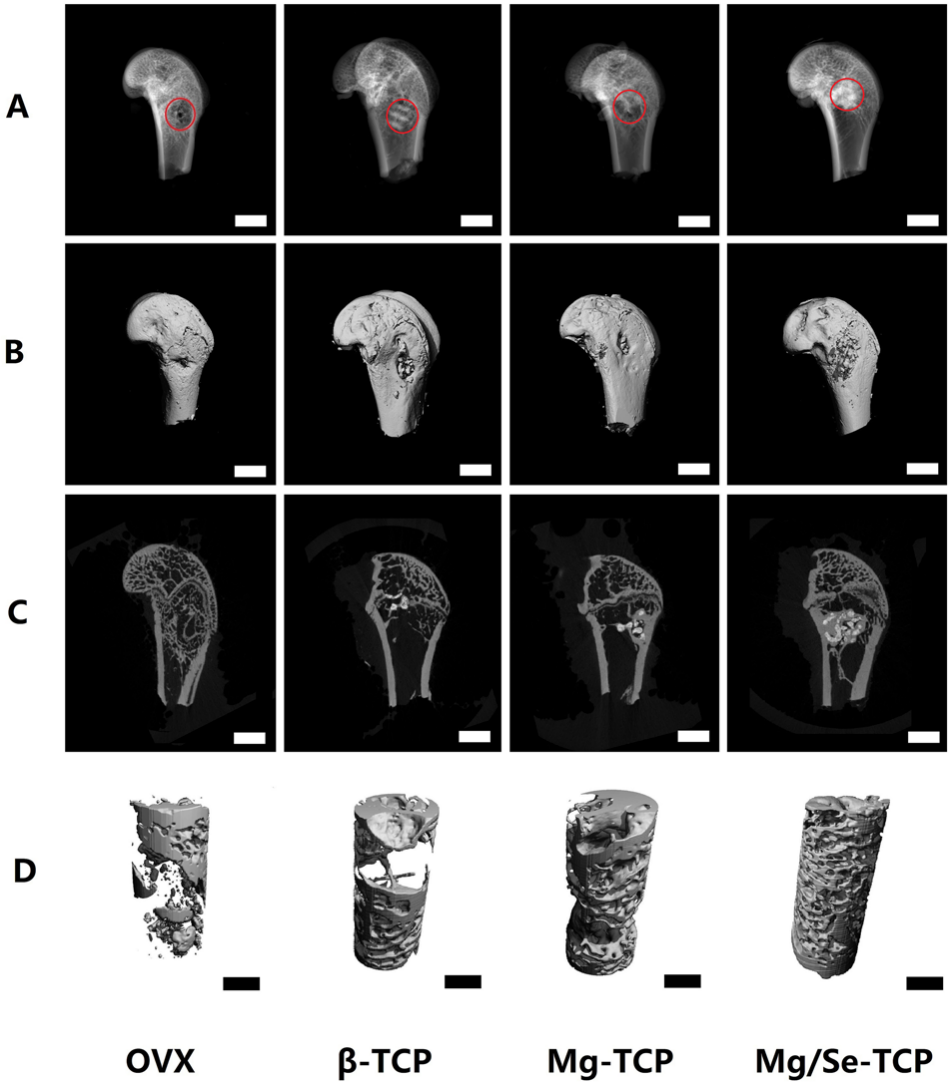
A X-ray detection; B Three-dimensional reconstruction of the overall view. C Three-dimensional CT scan; D 3D reconstruction of bone repair in the defect area
